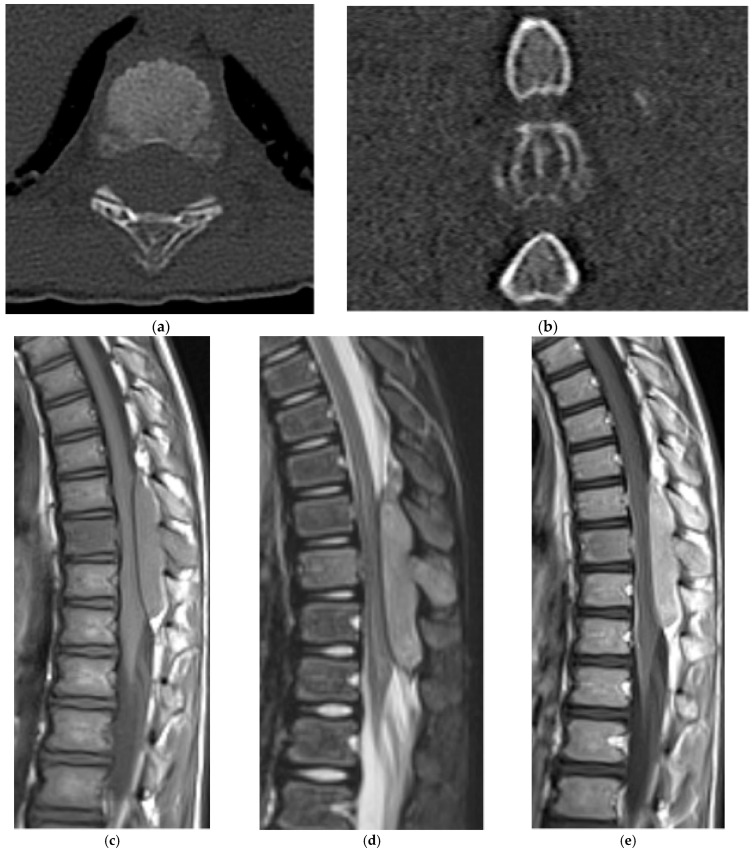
Ewing’s sarcoma. (a,b) Axial and coronal CT bone window, expansile lesion in the posterior elements of a lower thoracic vertebra with characteristic “onion skin” periosteal reaction. There is sclerosis of the vertebral body. (c) Sagittal T1 MR, intermediate signal intensity lesion involving the vertebral body with extension of the soft tissue component into the dorsal epidural space. (d) Sagittal T2 MR: lesion is mildly hyperintense. There is severe spinal canal narrowing with cord compression. (e) Sagittal T1 post-contrast MR, mildly increased enhancement.
